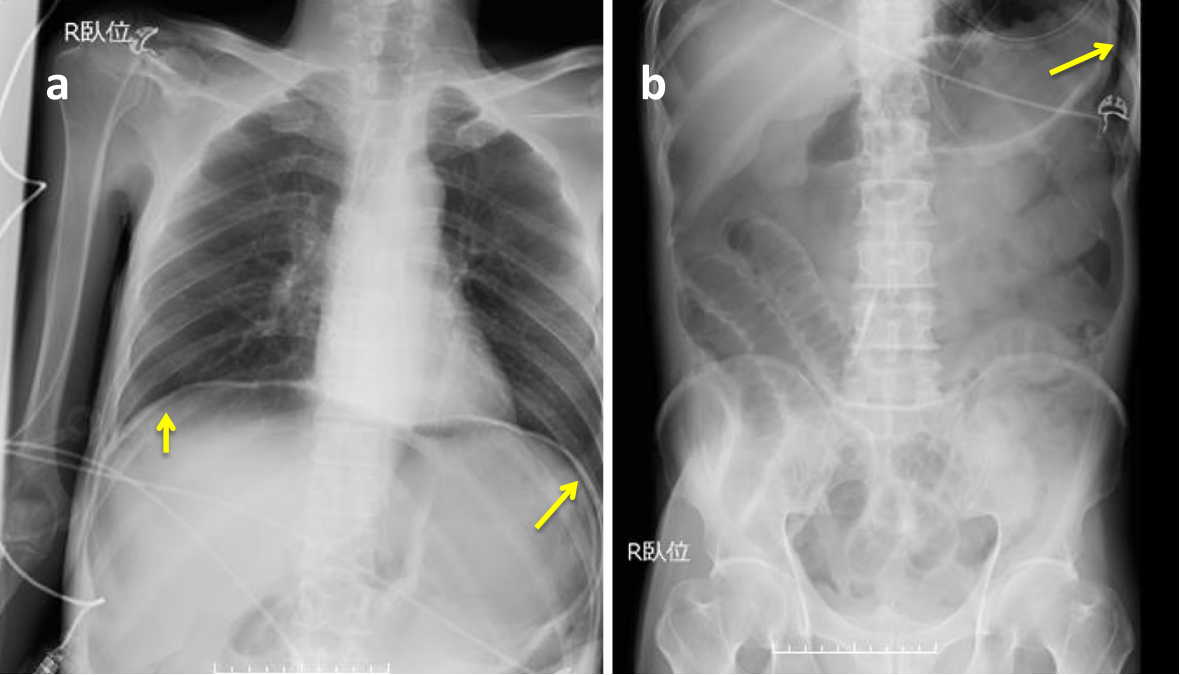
Chest (a) and abdominal (b) radiographs showing free air (*yellow arrows*)
